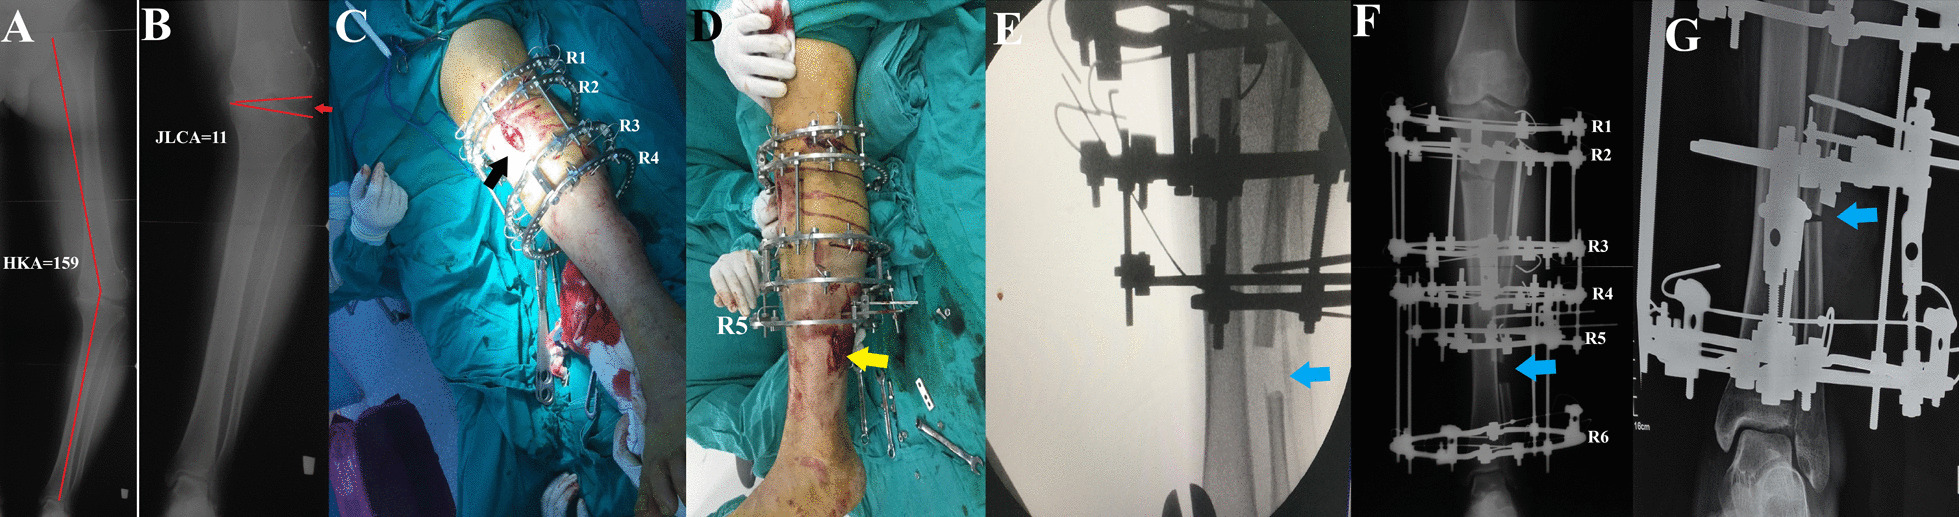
Male patient, 36 years old, presented with left knee pain and genu varum deformity. A preoperative long HKA radiograph showing varus deformity of 159°. B Joint line convergence angle of 11°. Intraoperative, C After applying the Ilizarov and performing the medial wedge opening high tibial osteotomy (black arrow). D After performing the fibular osteotomy (yellow arrow showing the incision site). E Intraoperative fluoroscopic image showing the site of the fibular osteotomy (blue arrow). F and G Follow-up radiographs showing closing of the fibular osteotomy gap (blue arrow) after gradual distalization of the proximal fibula
